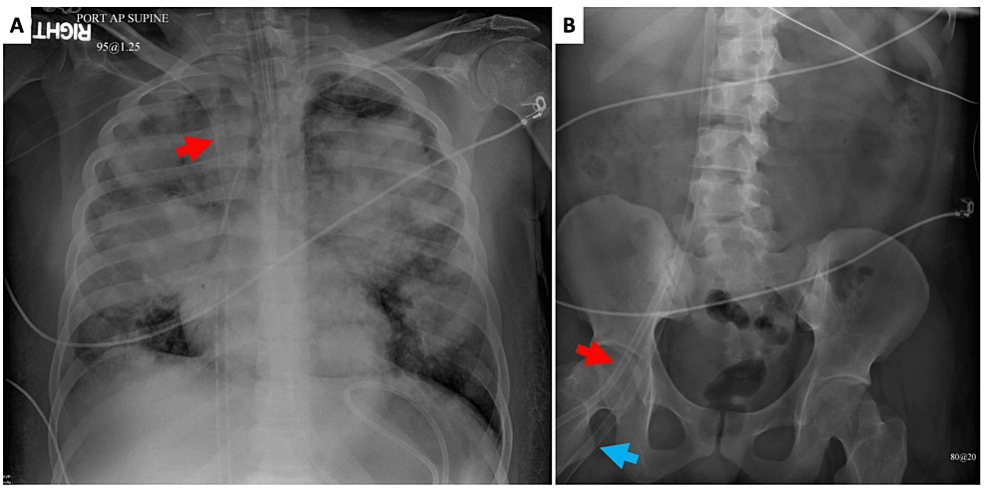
Chest (A) and abdominal (B) radiographs showing veno-arterial-venous ECMO configuration. Figure A: red arrow = 16-French right internal jugular return venous limb of the VAV-ECMO configuration. Figure B: red arrow = 17-French right femoral arterial cannula, blue arrow = 24-French right femoral venous drainage cannula. ECMO = extracorporeal membrane oxygenation; VAV-ECMO = veno-arterial-venous extracorporeal membrane oxygenation
